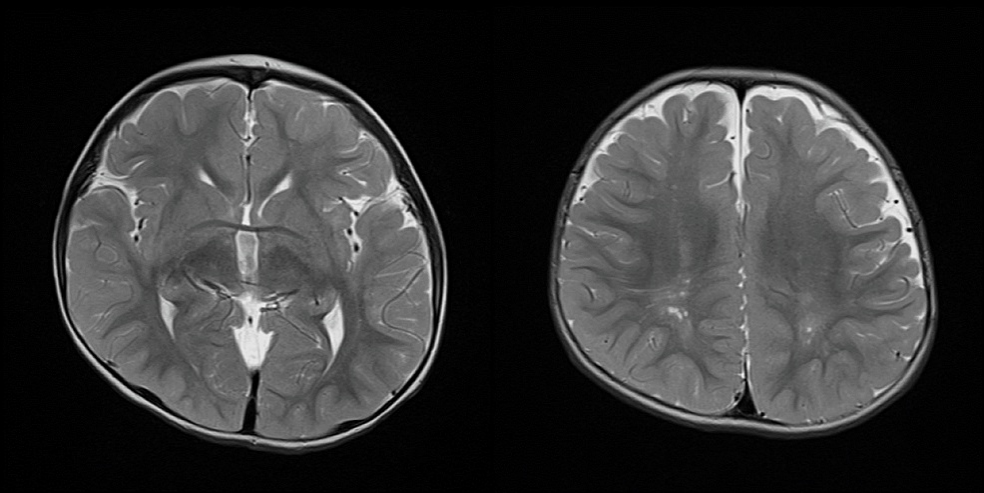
Head MRI T2WI findings at 1 year and 11 months old The image reveals high signal areas in the occipital lobe and deep frontal white matter. These signals are prominent in occipital lobe white matter and palely present in frontal lobe white matter. MRI: Magnetic resonance imaging; T2WI: T2 weighted image
